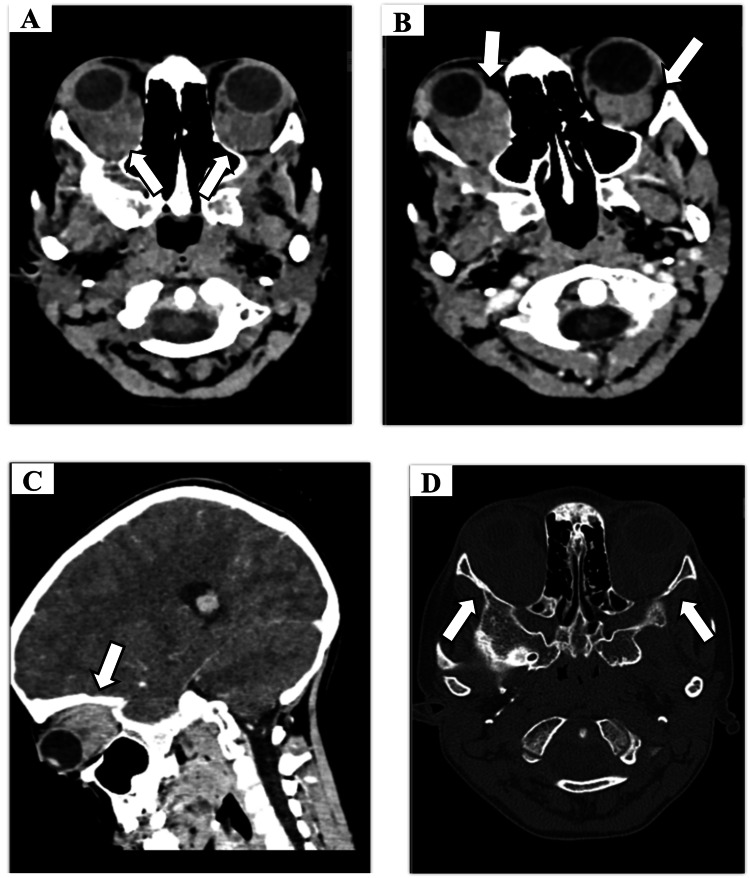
Cranio-orbital CT findings (A) Axial non-contrast CT image showing bilateral, ill-defined, isodense intraconal orbital infiltrates (arrows). (B) Axial and (C) sagittal post-contrast CT images demonstrating homogeneous enhancement of the infiltrates (arrows). (D) Axial bone window image confirming the absence of bone erosion of the orbital walls. CT: computed tomography
